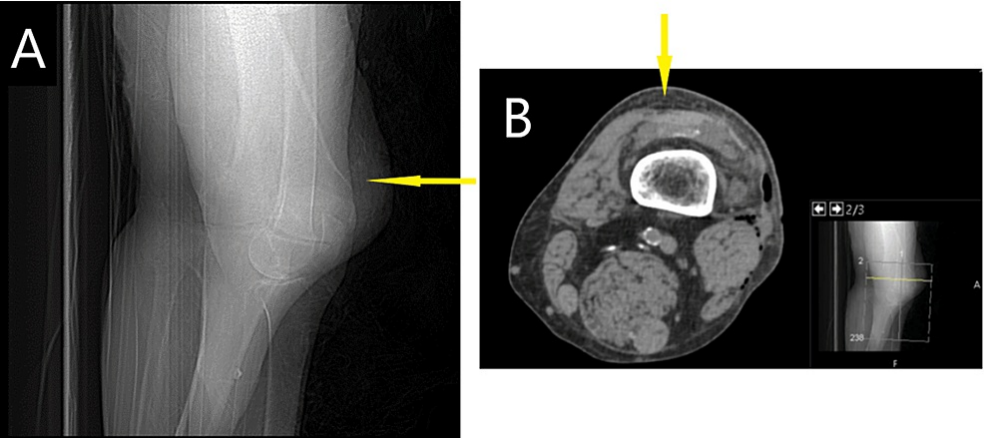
X-ray and CT scan without contrast of the left knee during the initial emergency department visit in January A) Initial X-ray showing a large amount of subcutaneous air with no evidence of acute bone or joint abnormalities. B) Initial CT showing broad anterolateral knee soft tissue defect with foci of superficial soft tissue gas.
